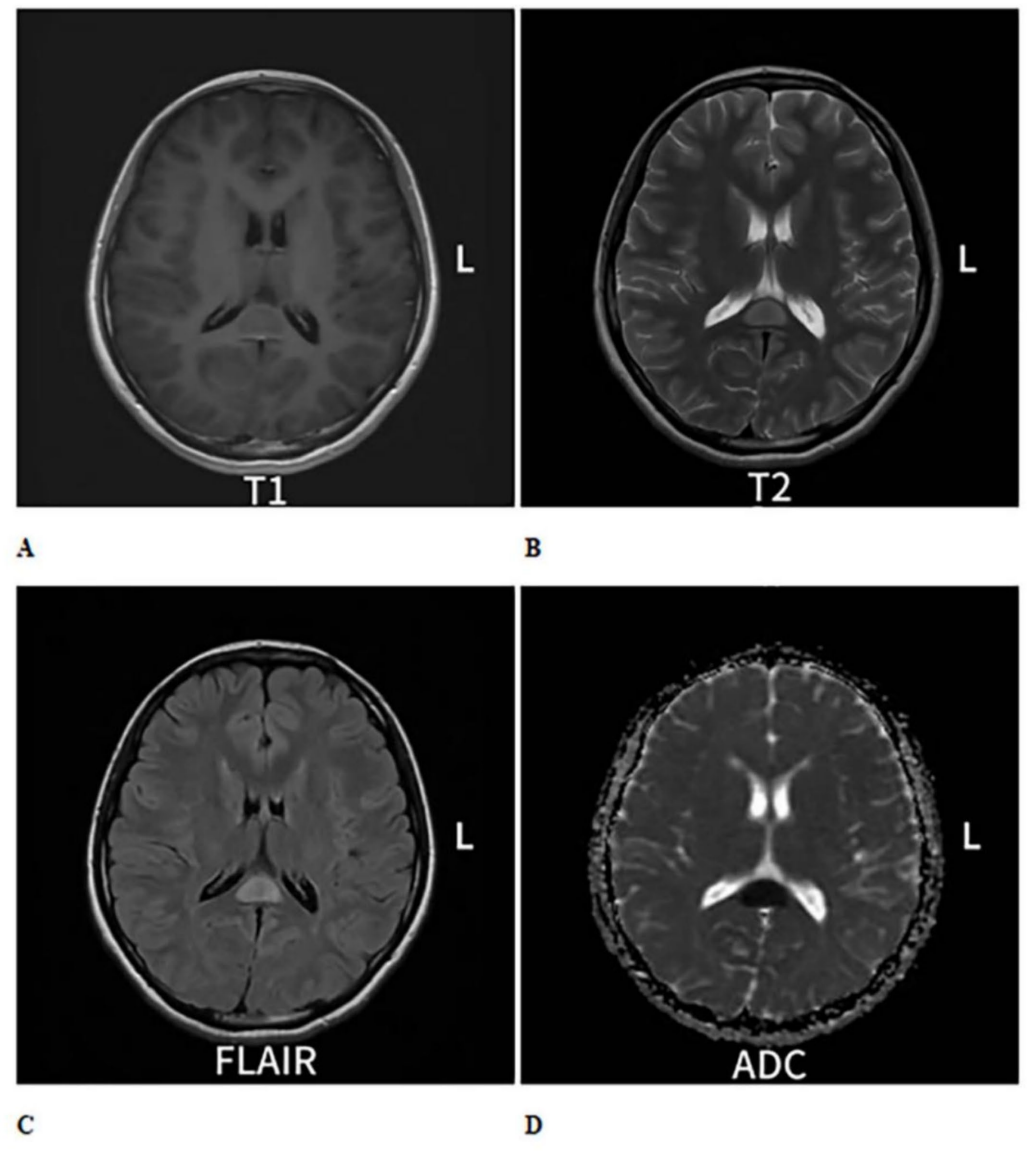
Cranial MRI plain scan with enhancement shows an oval-shaped abnormal signal in the midline area of the splenium of the corpus callosum. (A) On T1-weighted imaging (T1WI), it exhibits isointense to slightly hypointense signals (B), while on T2-weighted imaging (T2WI), it shows hyperintense signals. (C) On T2 fluid-attenuated inversion recovery (T2-FLAIR), the signal is also hyperintense. (D) Diffusion-weighted imaging (DWI) indicates restricted diffusion within the lesion, and no significant change is observed on enhanced scans.
